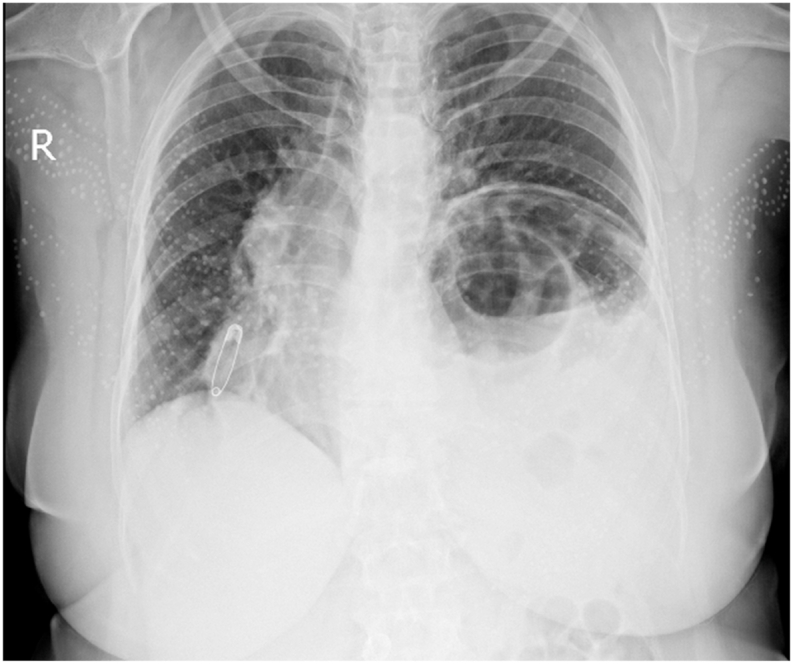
Plain PA chest x-ray in the first investigation side showing the entrance of splenic flexure of the colon into the left thoracic cavity besides mediastinal shifting and right inferior collapse of the right lung.
